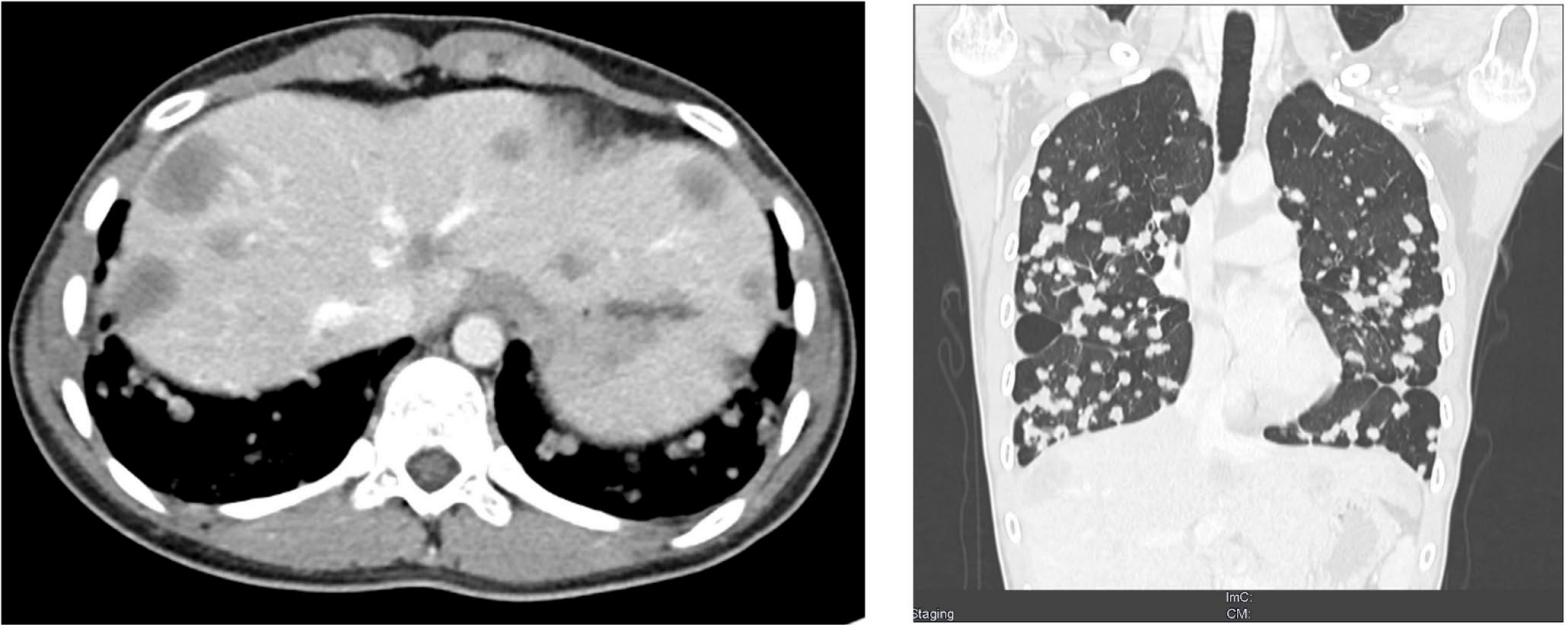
Baseline CT imaging of a patient with metastatic epithelioid hemangioendothelioma (EHE) involving liver and lungs
